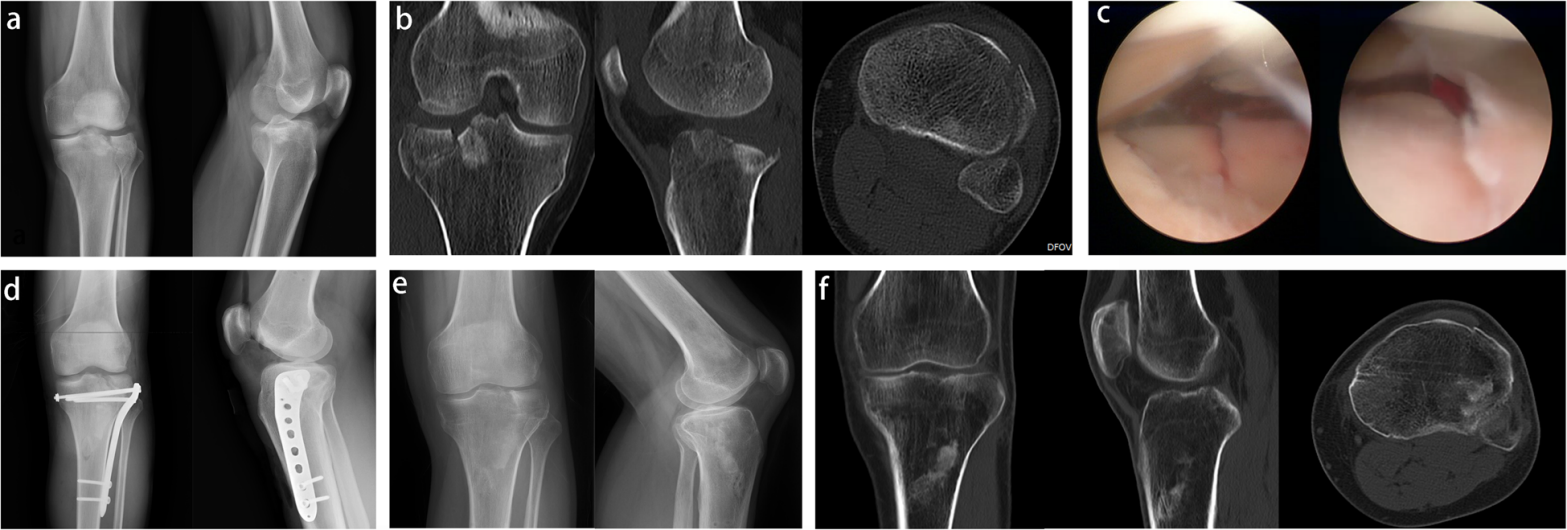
A 43-year-old male patient suffering from a Schatzker II fracture, treated with CRIF and arthroscopic examination. a Pre-operative radiograph. b Pre-operative CT scan. c Arthroscopic examination of the articular surface after reduction. d Post-operative radiographs immediately. e, f Post-operative radiographs and CT scan at the final follow-up
